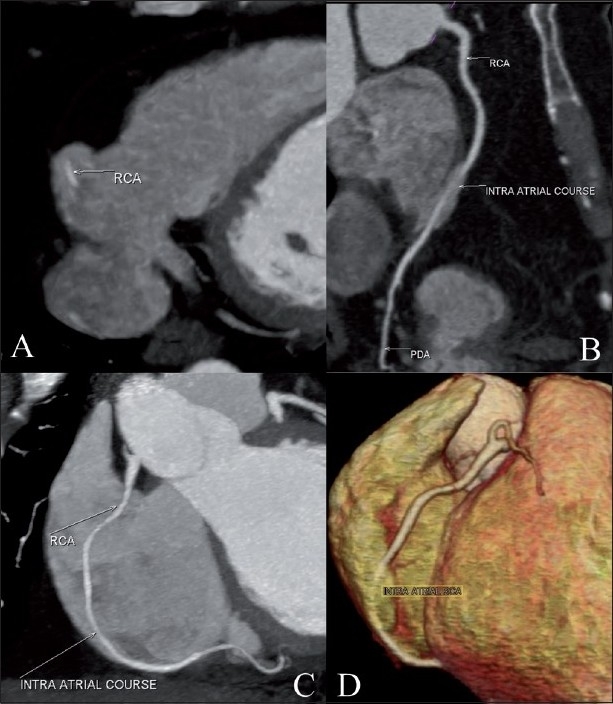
Cardiac CT. Axial maximum intensity projection (MIP) image (A) shows the intra-atrial location of the right coronary artery (RCA) (white arrow). The curved multiplanar reconstruction (B) and MIP (C) images show the normal origin and short intra-atrial course of the RCA (white arrows). The volume-rendered image (D) shows the entry and exit points of the RCA. Also, note the normal epicardial course of the proximal and distal RCA
